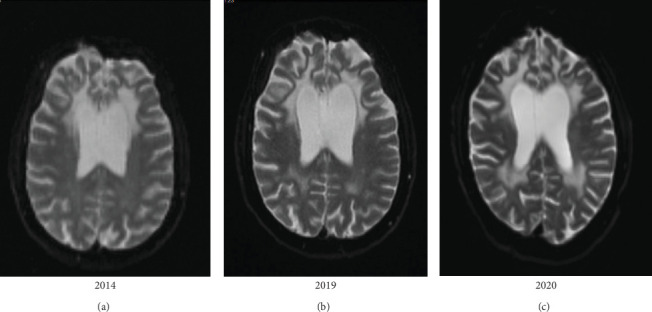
Axial T2-weighted MRI images showing progression of bilateral leukodystrophy white matter involvement in frontal and parieto-occipital lobes from 06/2014 (A) to 08/2019 (B) and 10/2020 (C).
